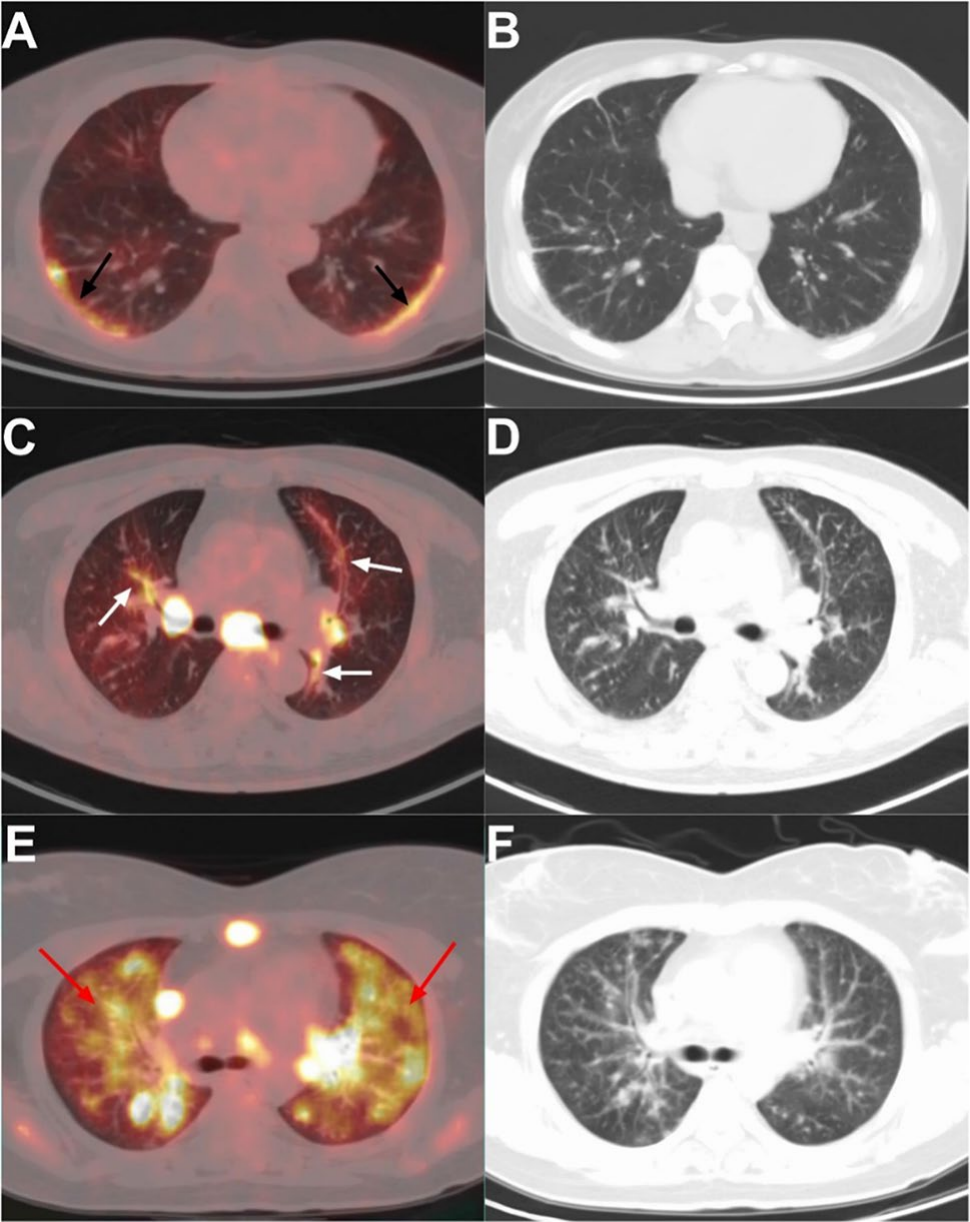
A, B, Axial fused 18F-FDG PET/CT image in a 55-year-old pancreatic cancer woman with pulmonary sarcoidosis showed subpleural hypermetabolism (black arrows; SUVmax, 5.3), and low-dose axial CT showed subpleural micronodules and pseudopleural plaques. C, D, Axial fused 18F-FDG PET/CT image in a 57-year-old gastric cancer man with pulmonary lymphangitic carcinomatosis showed Bronchovascular bundle hypermetabolism (white arrows; SUVmax, 4.0), and low-dose axial CT showed Bronchovascular bundle thickening. E, F, Axial fused 18F-FDG PET/CT image in a 31-year-old colon cancer man with pulmonary lymphangitic carcinomatosis showed lobar diffuse hypermetabolism (red arrows; SUVmax, 8.1), and low-dose axial CT showed multiple nodules and opacities in the lungs
